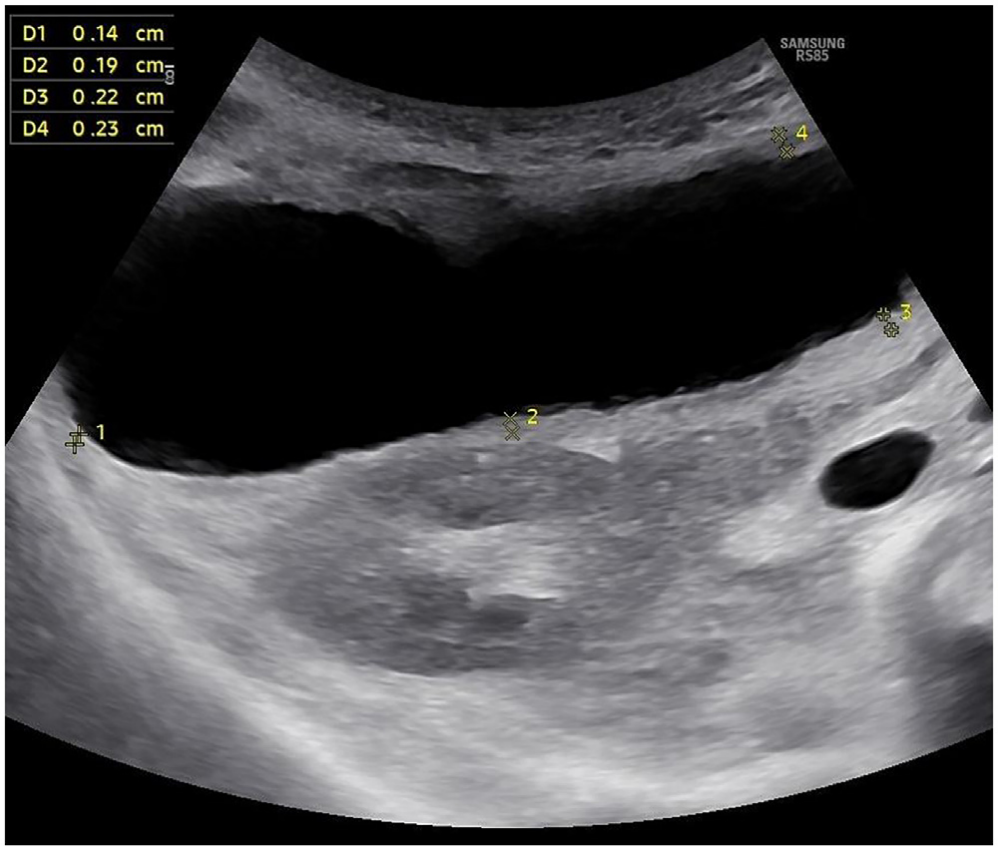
Ultrasound showing a distended gallbladder with normal wall thickness and no gallstones. Wall thickness is indicated in mm with yellow annotations.
